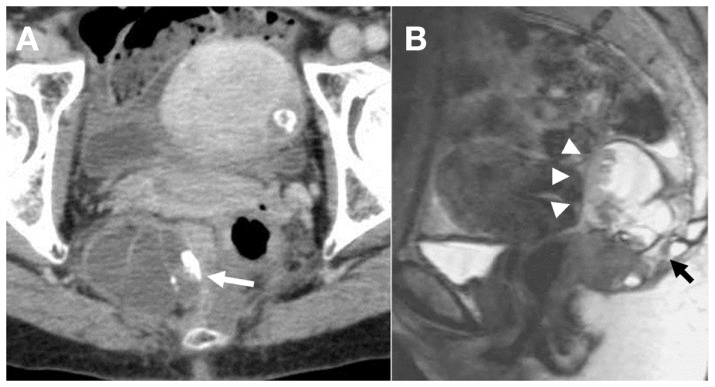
(A) Enhanced CT shows a presacral heterogeneous tumor, 67 mm × 45 mm, containing multiloculated cystic area and solid component with calcification (white arrow). (B) T2-weighted sagittal magnetic resonance image demonstrates a presacral non-enhanced cystic lesion with solid component, which was suspected to be strongly adhered to the rectal wall (white arrow heads), S5, and coccyx (black arrows).
